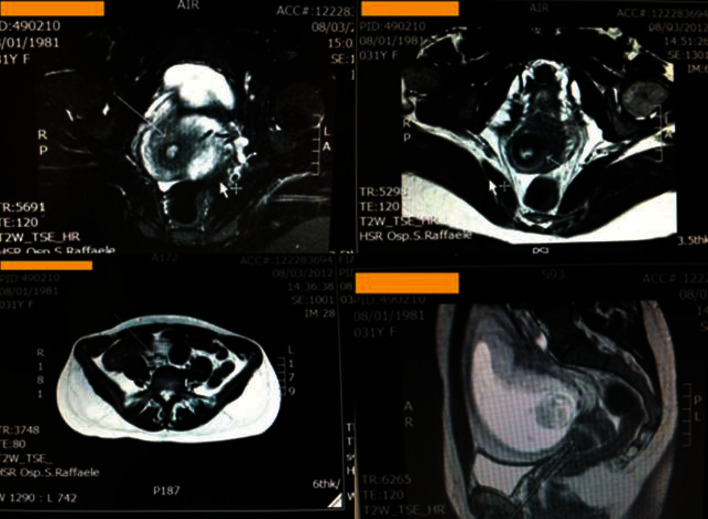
MRI mild-signal hyperintensity in the posterior-left and front cervical side (arrows); no aspects of infiltration of the parametrium.
